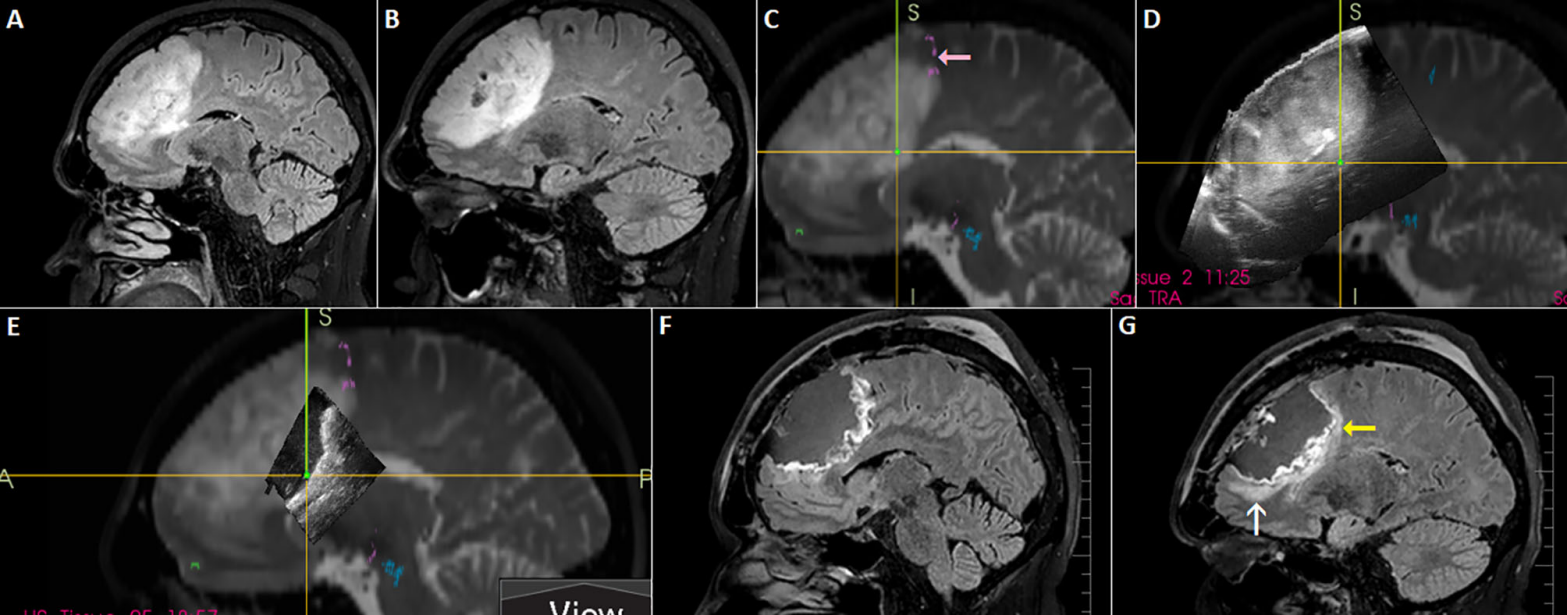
Left-sided frontal oligodendroglioma resected in a semi-sitting position. (A, B) Preoperative sagittal FLAIR MRI sequence (C) 3D T2 sequence fused with tractography. Note involvement of tracts originating in pre-supplemetary motor area (pink arrow) (D) 3D IOUS image fused with navigation MRI (E) Incomplete filling of the resection cavity with saline due to non-horizontal placement of the craniotomy resulted in insufficient scanning of anterior part of the resection cavity (F, G) Postoperative sagittal FLAIR MRI sequence showing resectable residuum (white arrow), as well as residual tumor involving eloquent tracts (yellow arrow). 3D, three-dimensional; FLAIR, fluid attenuated inversion recovery; MRI, magnetic resonance imaging; IOUS, intraoperative ultrasound.
